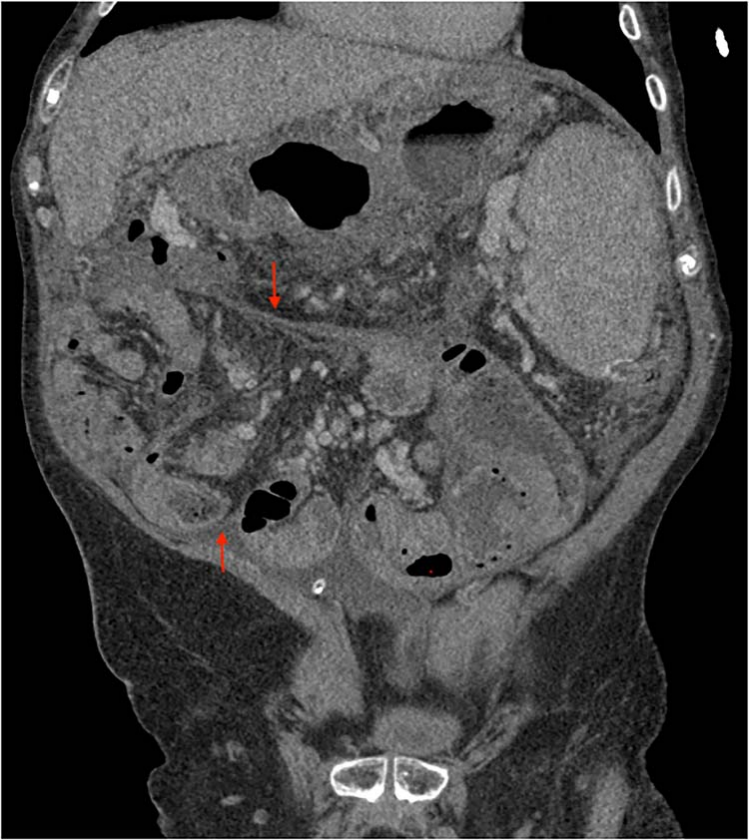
Portal phase coronal section image on CT showing loops wrapped in a membrane-like structure (arrow).
